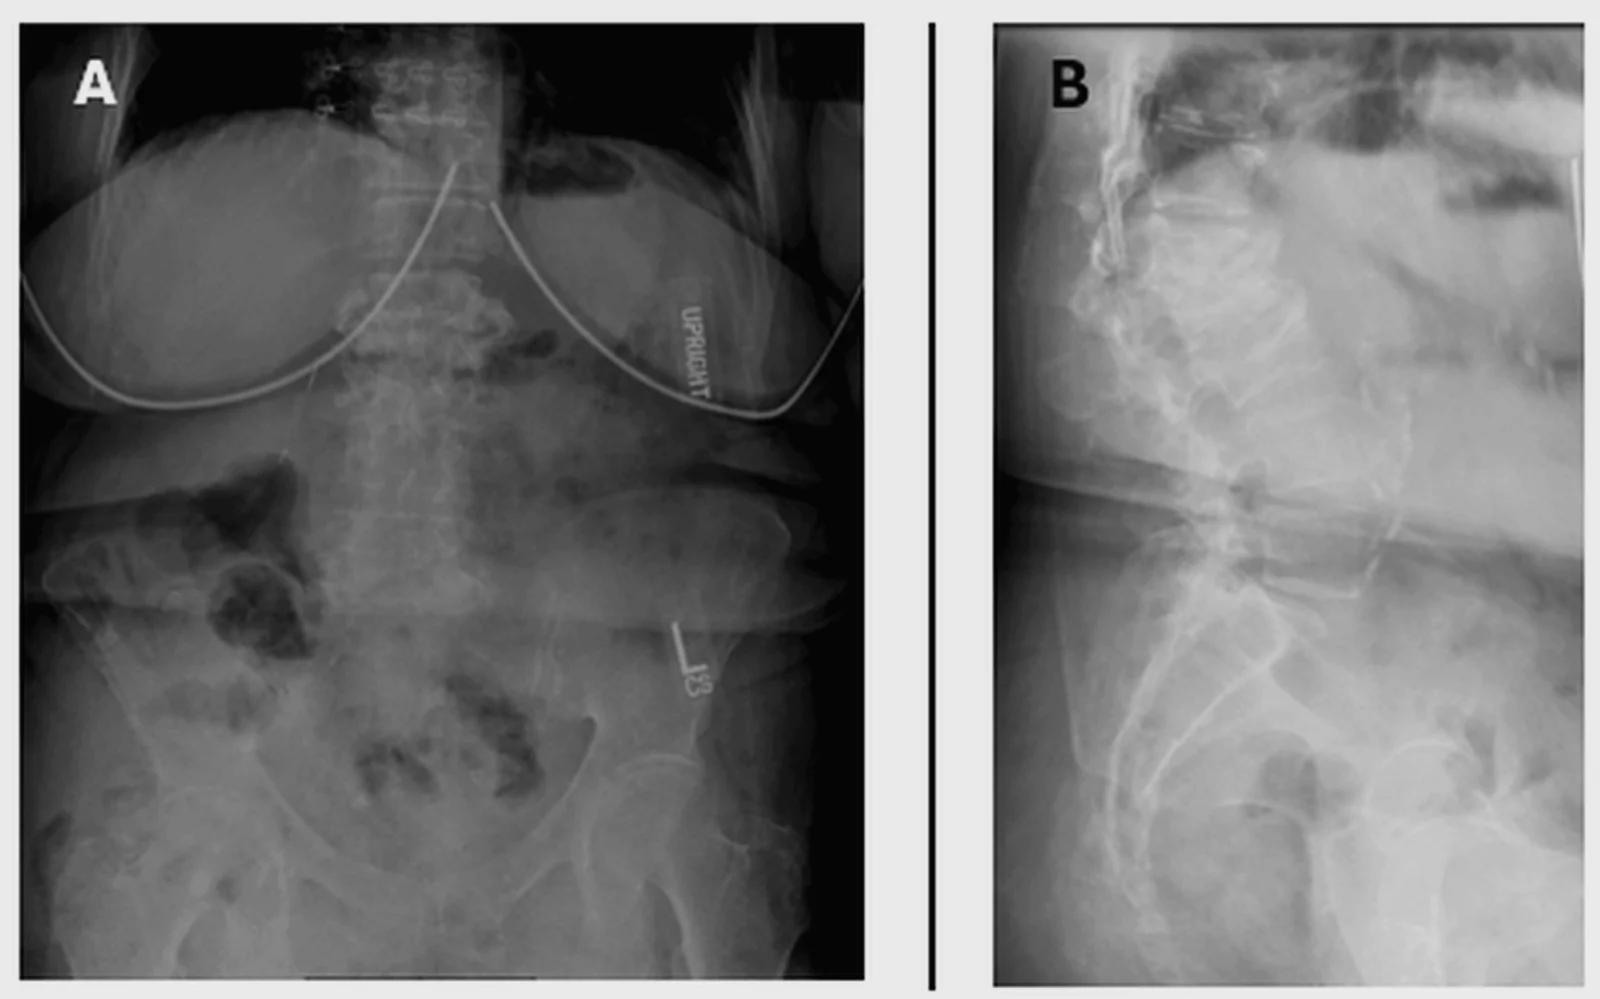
(A) Preoperative standing anteroposterior radiograph and (B) lateral radiograph of the lumbar spine after removal of the prior L2-L5 instrumentation, demonstrating absence of hardware and progressive kyphotic alignment.
